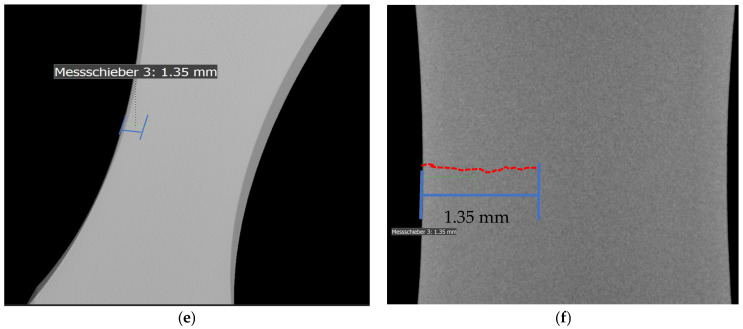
Non-destructive analysis applied to oxidized sample after fatigue testing: (a,b) liquid penetrant inspection, and (c) overview of CT examination: detail volume image—radius area, (d) microcrack measurement in radius area, (e) overview of CT examination: detail volume image-frontal surface of sample, and (f) microcrack measurement in the frontal surface area. The red line indicates the microcrack length, while the blue line represents the measured value obtained during the analysis.
